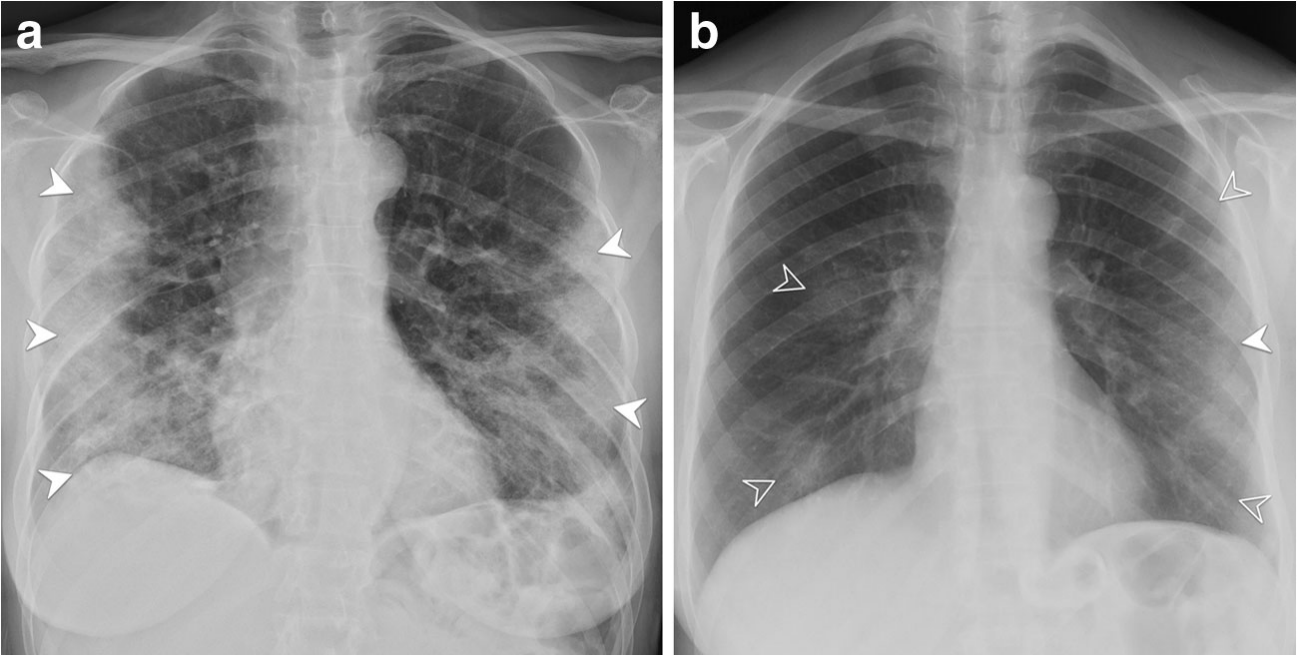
Chest X-ray (CXR) findings at the emergency department presentation in two patients with confirmed COVID-19 and opposite outcomes. a CXR shows bilateral, mostly peripheral, ground-glass opacities (GGOs) admixed with consolidation (consolidation-predominant) (arrowheads). Reviewer 1 assigned a *Brixia* score of 14 and a percentage of lung involvement of 60%. Reviewer 2 assigned a *Brixia* score of 15 and a percentage of lung involvement of 50%. This patient had a prolonged stay in the intensive care unit and died 11 days after presenting to the emergency department. b CXR shows bilateral GGOs, either pure (empty arrowheads) or admixed with consolidation (GGO-predominant) (solid arrowhead). Reviewer 1 assigned a *Brixia* score of 6 and a percentage of lung involvement of 30%. Reviewer 2 assigned a *Brixia* score of 5 and a percentage of lung involvement of 25%. This patient was discharged from the emergency department after a short-term observation with home care and isolation precautions and was alive at the end of the study period
